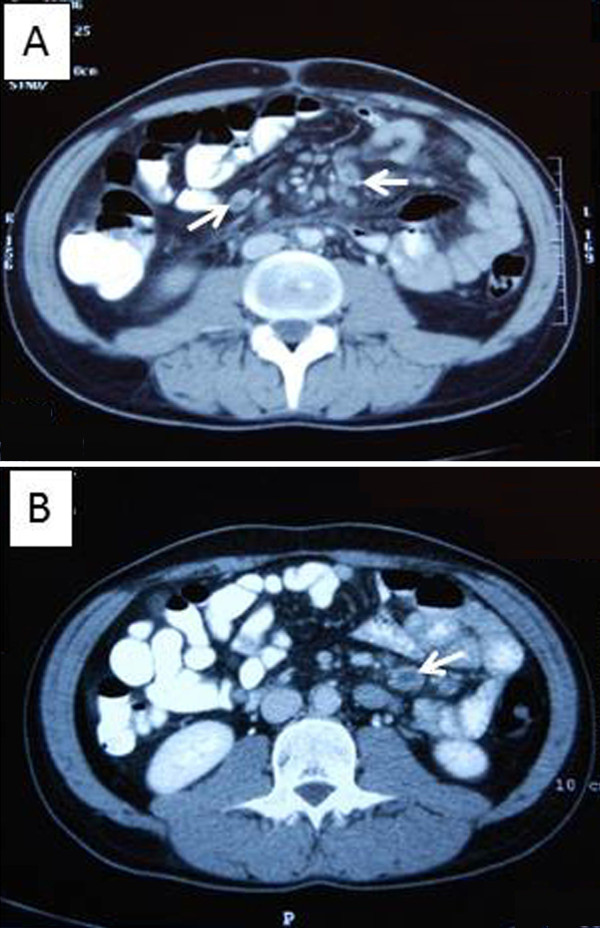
Computed tomography scan of the patient’s abdomen (A and B): A mesenteric lymphadenopathy of 2.7cm maximum diameter with a hypodense center was detected (white arrows)
